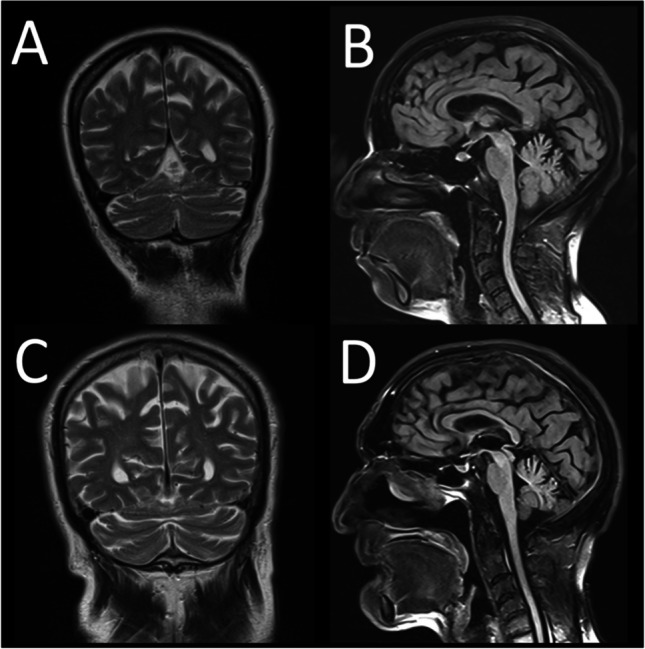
A, B Brain MRI image of patient #19 (sagittal and coronal). C, D Brain MRI image of patient #20 (sagittal and coronal). Diffuse cerebral and cerebellar atrophy were present in both patients
